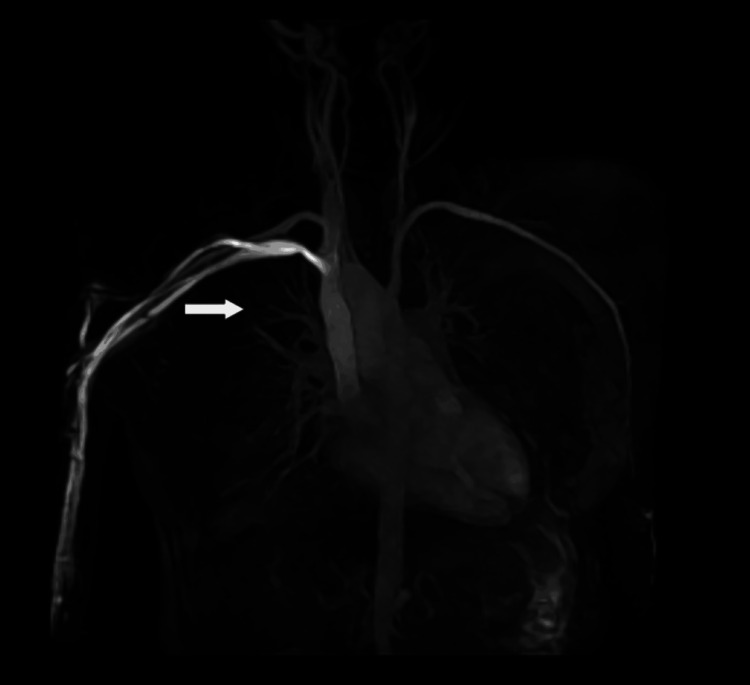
Contrast-enhanced chest magnetic resonance image Contrast-enhanced chest magnetic resonance image showing that the pulmonary arteriovenous malformation has disappeared.
